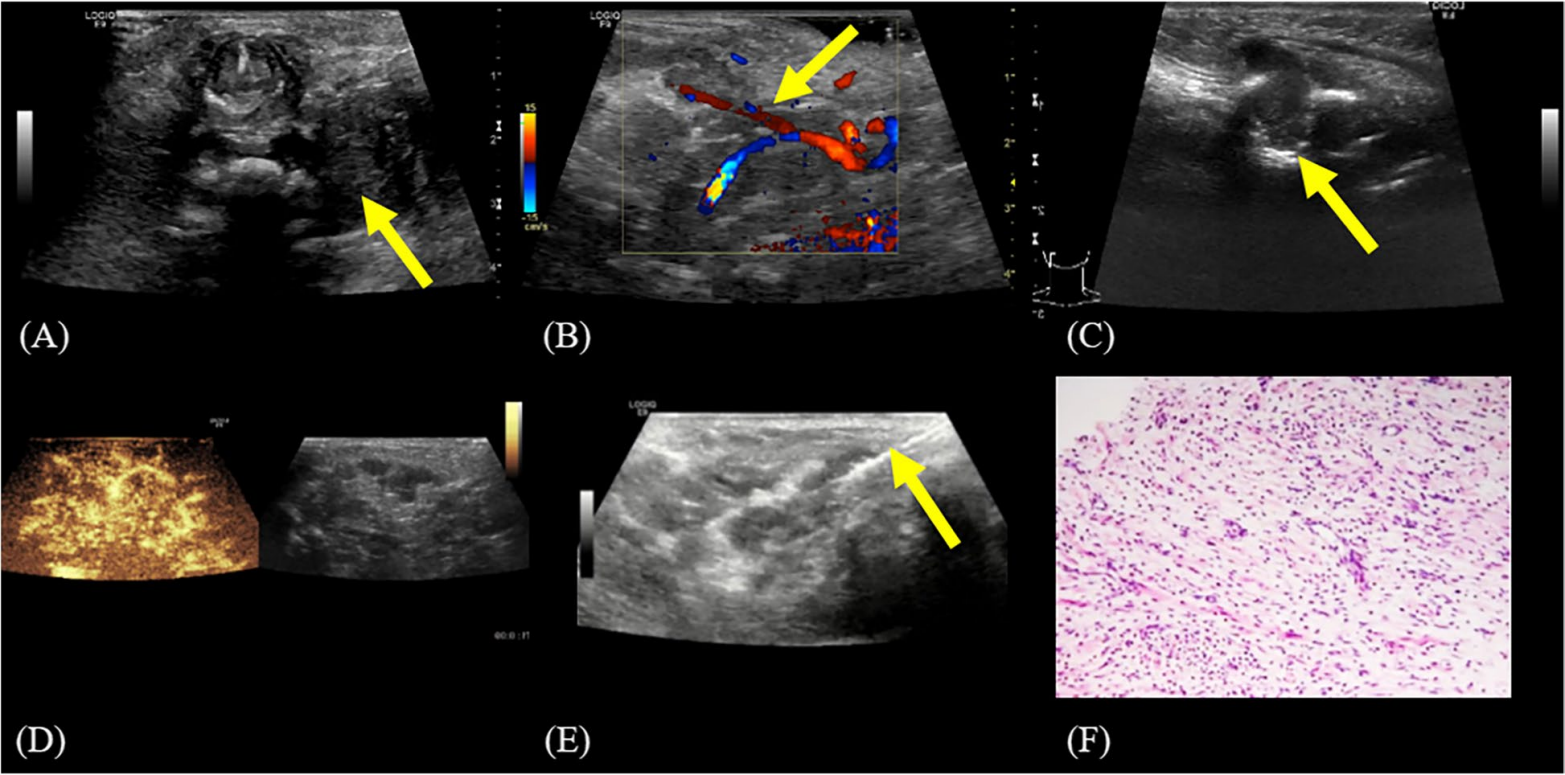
Ultrasound and pathological images. A Transverse image of the irregular hypoechoic mass in the deep facial space beside the left cervical spine (arrow); B Image of the mass shows bead-like echo, encircling the external carotid artery and its branches; C Bone destruction is observed in the left mandible (arrow); D Contrast-enhanced ultrasound shows rapid hyperenhancement and slow clearance within the mass, without any obvious non-enhancement area; E Ultrasound-guided puncture of the mass (arrow); F Puncture tissues mainly consist of spindle cells
